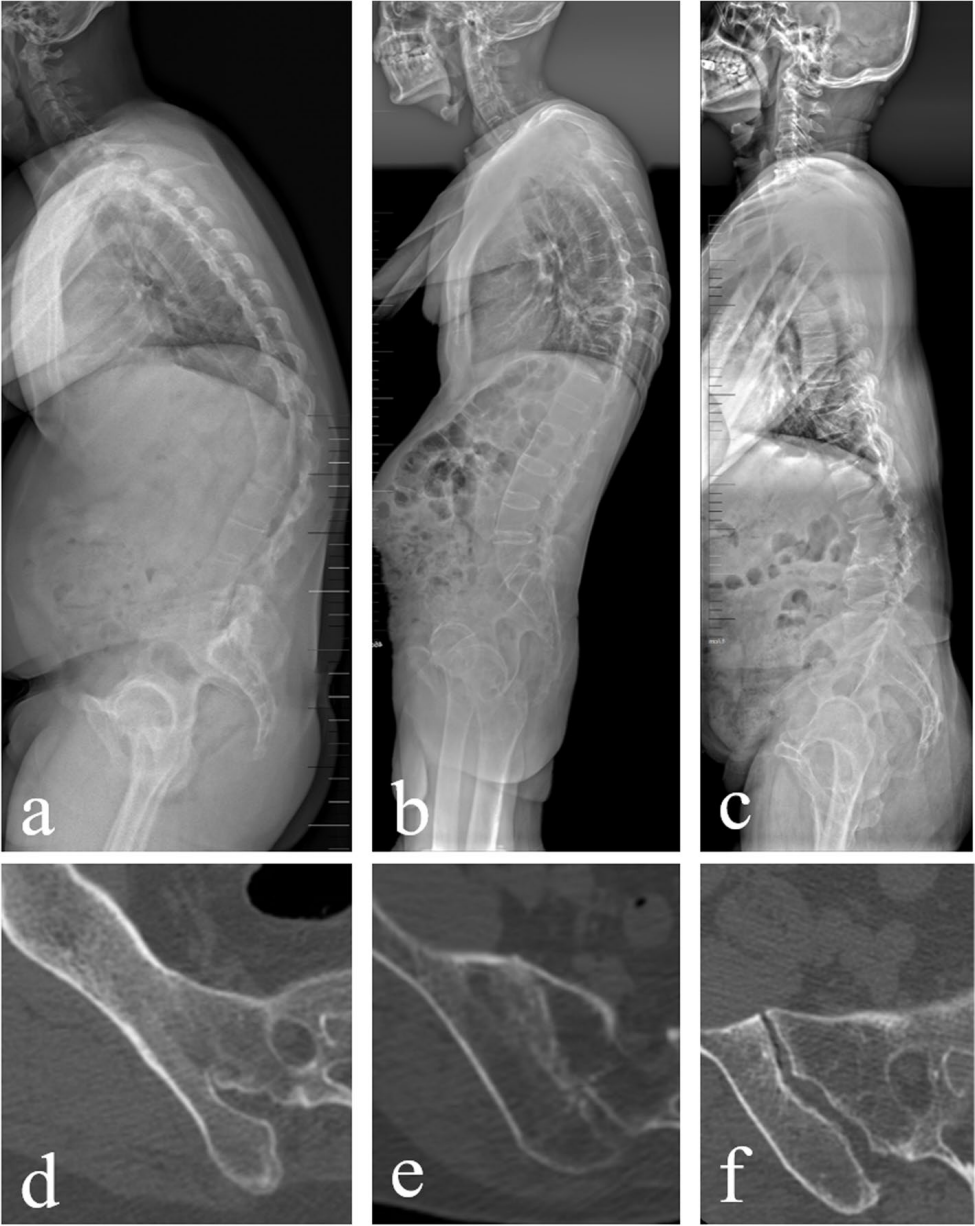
Standing lateral radiograph and CT of sacroiliac joint. a AS patient with thoracolumbar kyphosis (GK: 77°). b Non-deformity patient with AS. c Sagittal deformity patients without AS (posttraumatic kyphotic deformity in thoracolumbar spine). d-e CT scans have shown bony fusion in sacroiliac joints of the deformity patient with AS, also the non-deformity patient with AS. f No erosion or ankylosis was found in sacroiliac joints of sagittal deformity patients without AS
